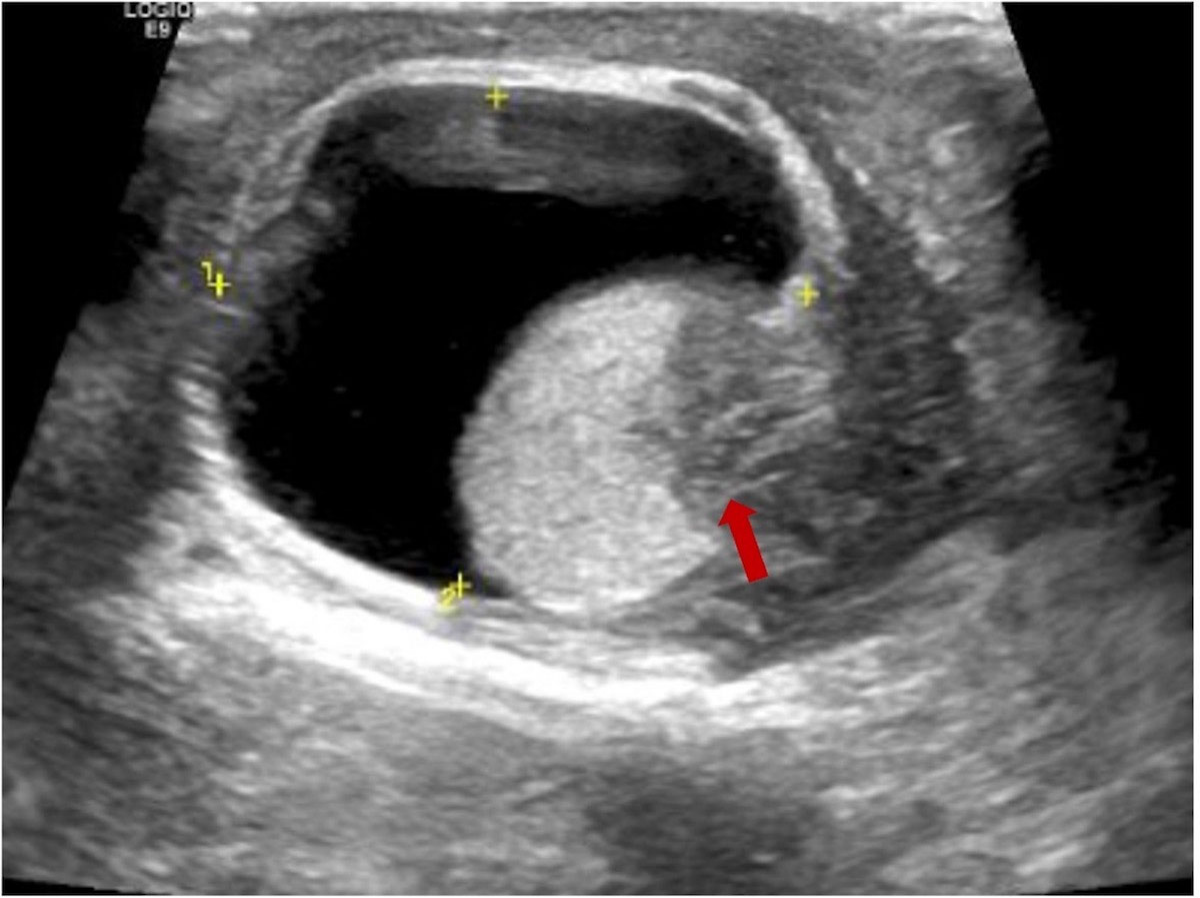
Scrotal ultrasound suggests inflammatory changes in the left epididymis and low echogenicity (red arrow) in the lower part of the left testicle.
